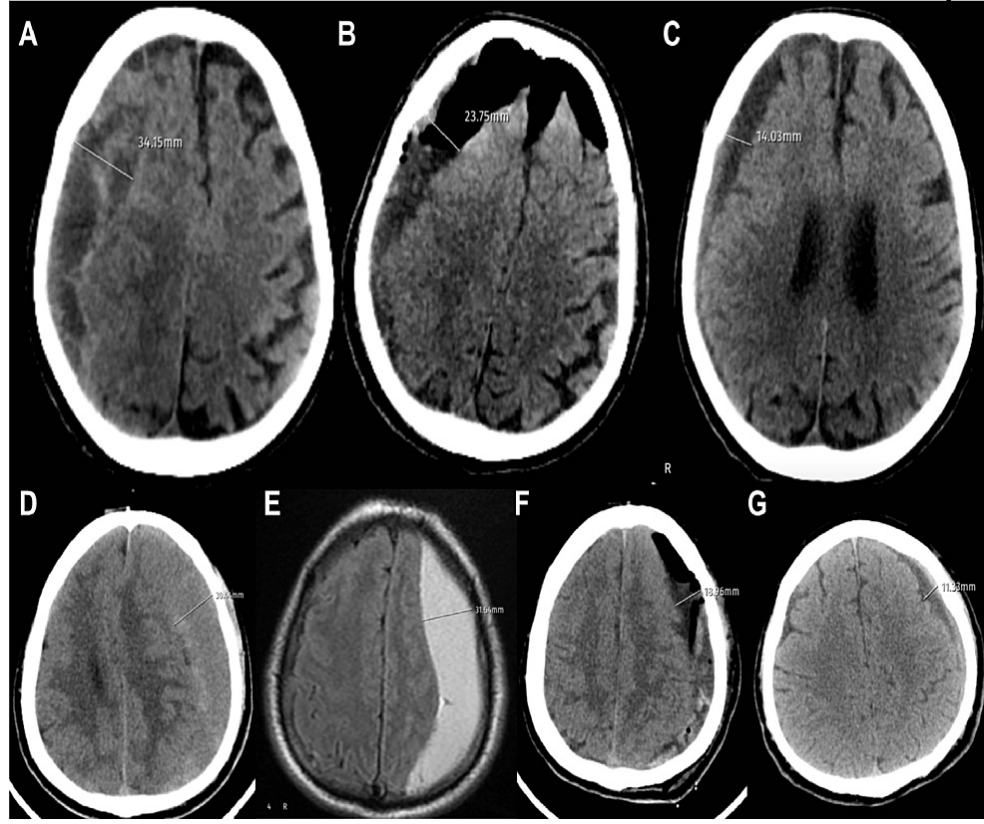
Examples of measurement of subdural hematoma sizes Right chronic subdural hematoma containing septa: A. preoperative CT image, B. early postoperative CT image, C. postoperative second-week image, D. preoperative CT image of chronic subdural hematoma in subacute formation on the left side, E. preoperative MRI image, F. early postoperative CT image, and G. postoperative second-week CT image. CT: computed tomography, MRI: magnetic resonance imaging
